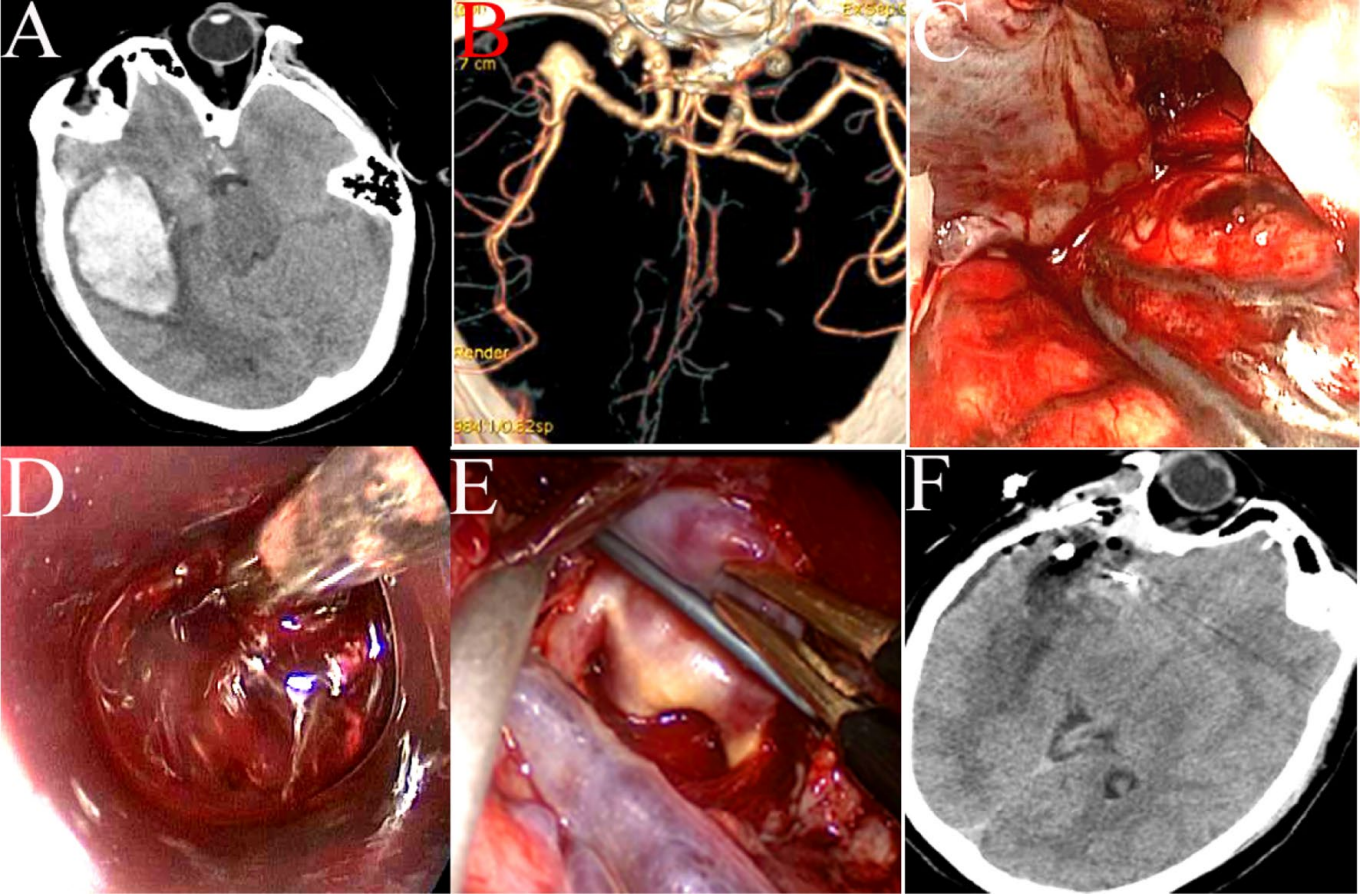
A ruptured MCA aneurysm with a hematoma was clipped under neuroendoscopy. (A) Preoperative CT showed a large hematoma in the right temporal lobe. (B) Preoperative CTA showed a saccular aneurysm at the right MCA bifurcation. (C) The brain pressure was high after opening the dura. (D) The hematoma was evacuated under neuroendoscopy. (E) The aneurysm was exposed and clipped under neuroendoscopy. (F) Postoperative CT showed complete removal of the hematoma and proper placement of the clip.
